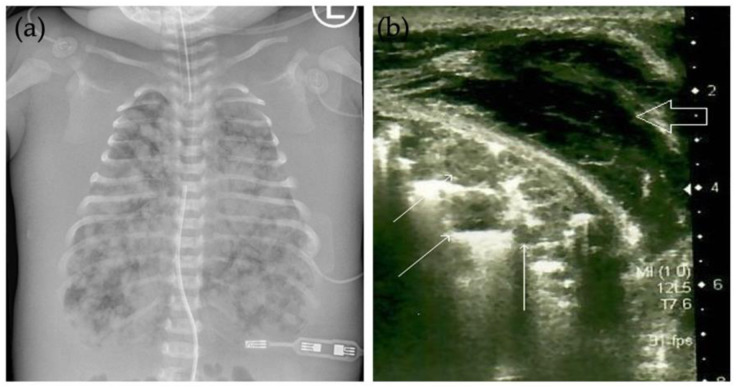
Frontal radiograph and ultrasonography of the lung. (a) Frontal chest radiograph shows multiple round lesions of different sizes diffusely involving the entire lung. Generalised body oedema in keeping with hydrops fetalis can be seen. Also noted are endotracheal tube, nasogastric tube, and umbilical venous line. (b) Ultrasonography of the lung showed multiple round hypoechoic solid nodules within the lung parenchyma (thin white arrows). No pleural effusion was seen. Severe body oedema is also noted (thick void arrow).
